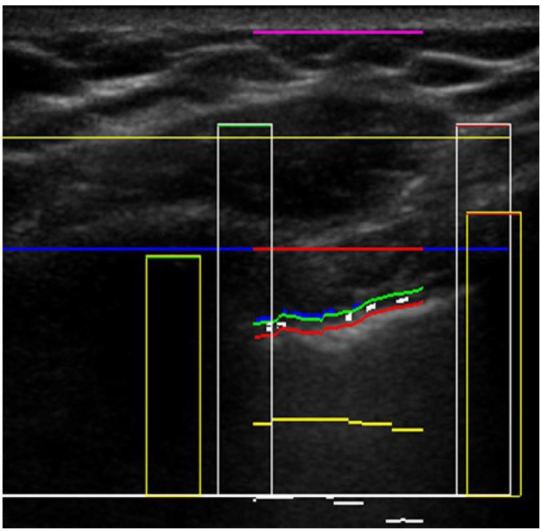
In this B-mode image, the intelligent focused assessment with sonography for trauma (iFAST) has correctly identified the pleural line in order to examine for sliding lung sign. The purple line denotes the skin surface. The first horizontal yellow line is the pectoralis muscle. To find the pleural line, the iFAST first locates the rib shadows (yellow rectangles). The red break in the blue horizontal line between the ribs defines the intercostal space. The pleural line appears like a road with paired green and red horizontal lines in the intercostal space. The small white rectangles on the “road” denote pixel movements back and forth along the pleural line, indicating normal sliding lung.
